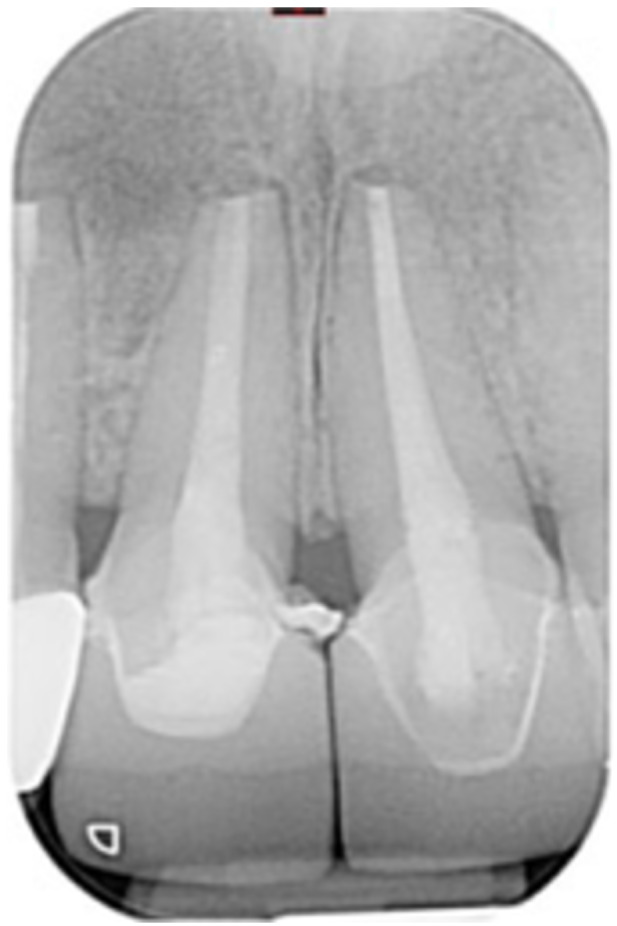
Case 4: The preoperative CBCT and periapical radiograph show an extensive periapical radiolucency associated with tooth 21. Apical surgery was performed on tooth 21 after the completion of a satisfactory nonsurgical root canal treatment on the tooth. At 6 months, marked healing can be seen, evidenced by a reduction in the periapical radiolucency size associated with tooth 21, with almost complete healing noted. Note that the crown on tooth 21 has also been replaced with a new one. Cases 3 and 4 are from the same patient but with different L‐PRF preparations.
